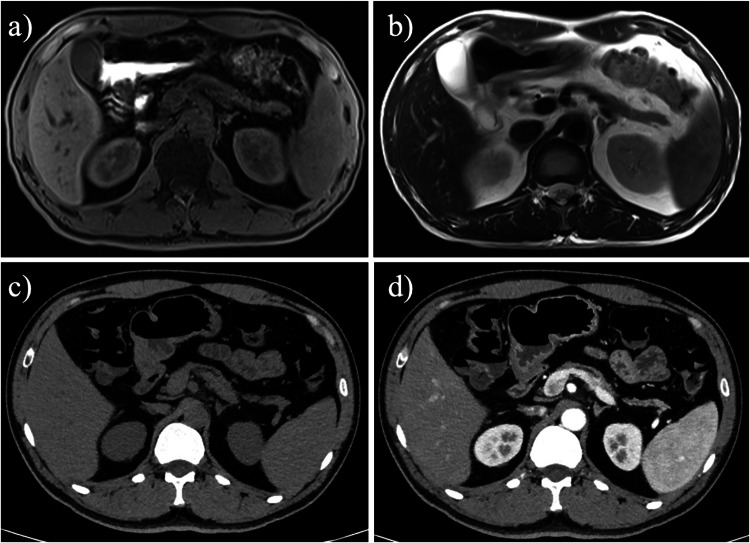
A 33-year-old man with chronic pancreatitis and pancreatic atrophy. Axial T1-weighted fat-suppressed MR image (a), T2-weighted MR image (b), axial unenhanced computed tomography (CT) image (c), and contrast-enhanced CT image (d) demonstrated thread-like pancreatic morphology with marked volume reduction
